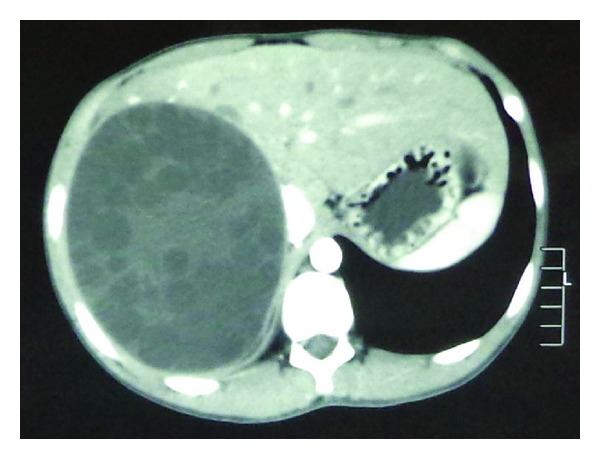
Computed tomographic scan of liver showing hydatid cyst.
